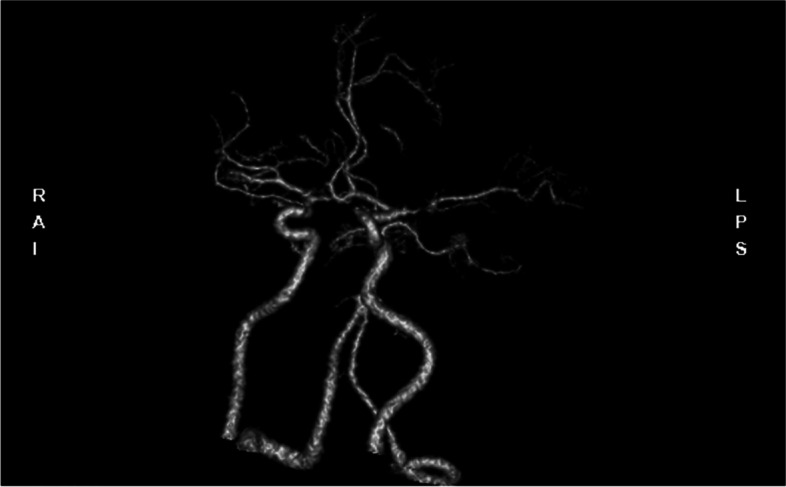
CTA imaging of the patient. It shows that severe stenosis of the MI segment of the middle cerebral artery
